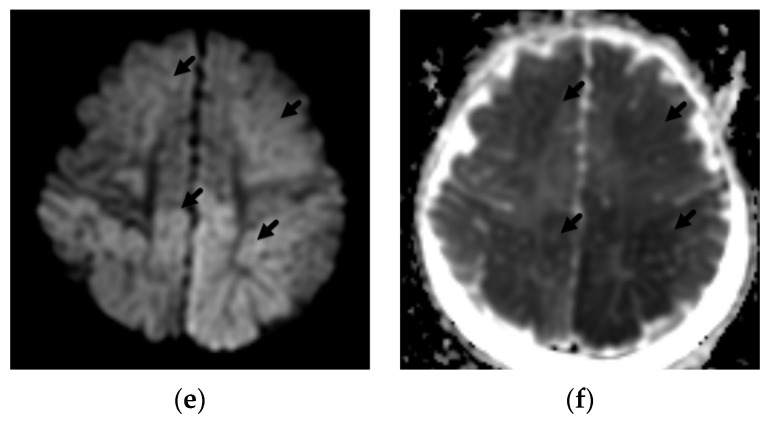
Diffuse, bilateral hemispheric attenuation abnormality in a 3-month-old male presenting in status epilepticus with multifocal facial, extremity, and trunk bruises. (a) Initial axial noncontrast head CT image with diffuse, abnormal attenuation throughout both cerebral hemispheres (white arrowheads); (b) coronal noncontrast CT image shows thin subdural hemorrhage along the left tentorial leaflet (white arrow); (c) coronal reconstruction bone algorithm image from an abdominal CT at presentation shows healing, bilateral posterior rib fractures (black arrowheads); (d) axial T2-weighted image on day 4 demonstrates diffuse, hemispheric loss of gray-white matter differentiation (black arrowheads); (e) axial b-1000 diffusion-weighted image demonstrates diffuse areas of abnormal, restricted diffusion (black arrows) in both cerebral hemispheres consistent with cytotoxic edema/hypoxic-ischemic injury; (f) apparent diffusion coefficient image shows corresponding regions of diffusion restriction predominantly in cortical and subcortical regions of both cerebral hemispheres (black arrows).
